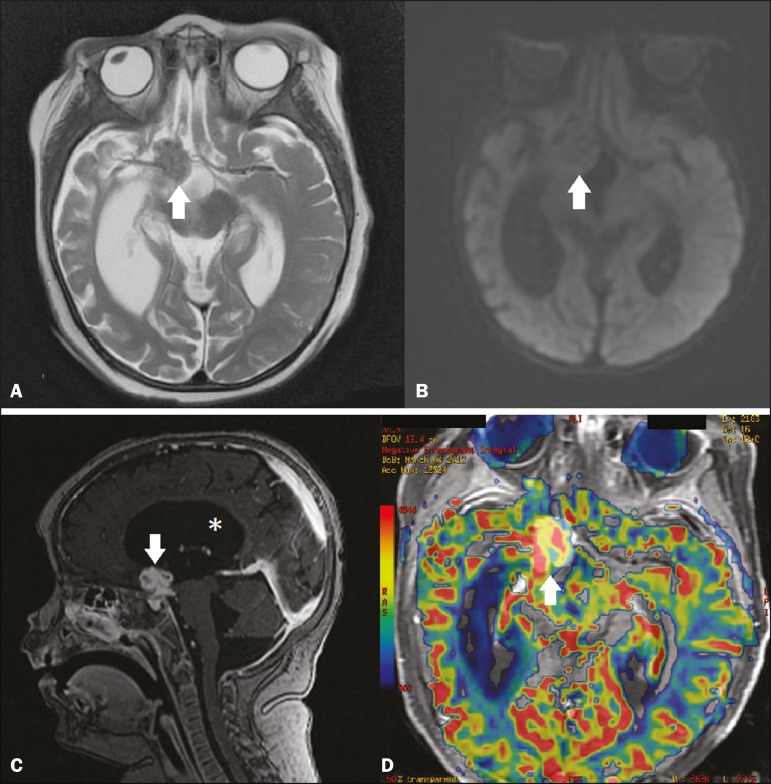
MRI. A: Axial T2-weighted sequence, showing a suprasellar lesion
